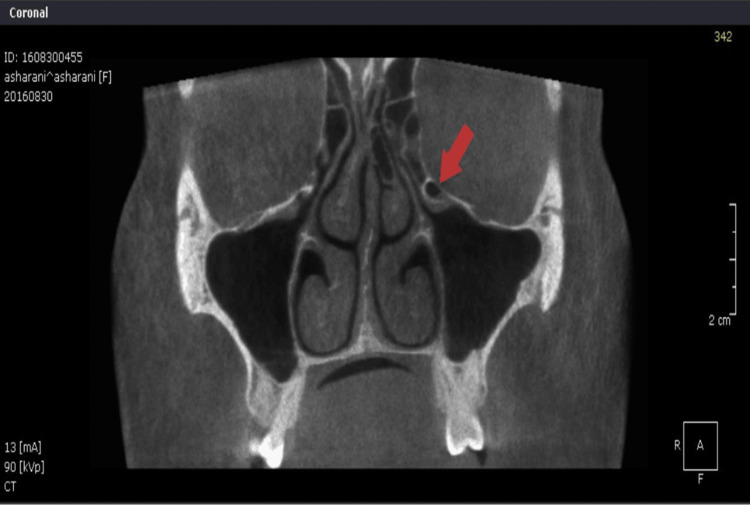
Haller cell (CBCT: coronal section)
